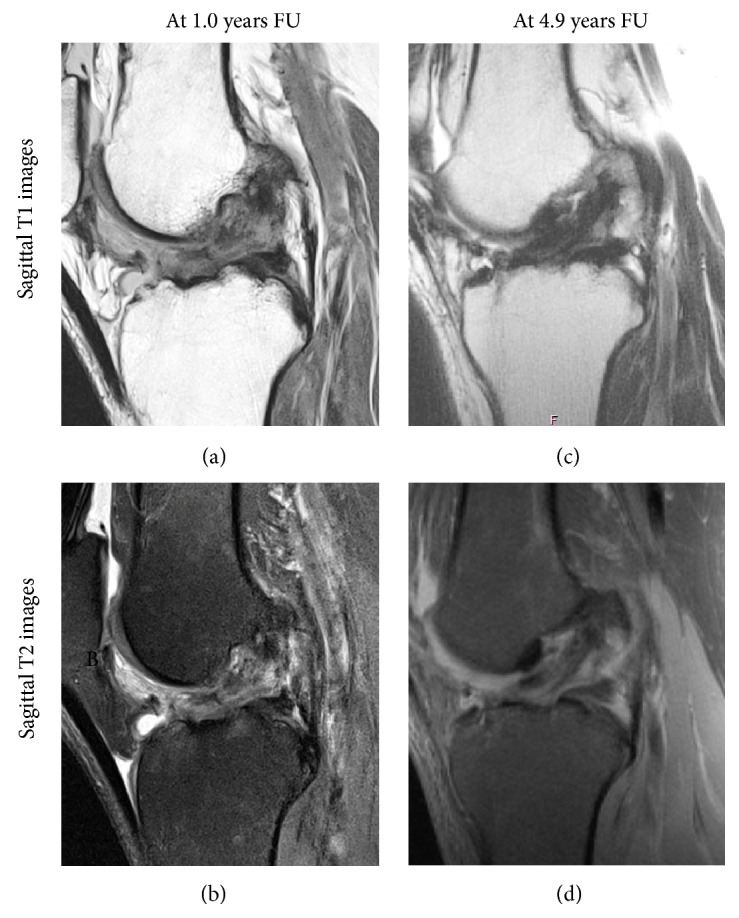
Sagittal T1 (upper row) and T2 (lower row) MR images are shown of the same patient at one and five years of follow-up. At one-year follow-up, the ligament is hyperintense and even appears to have a partial rupture of the ligament. This patient was clinically stable, and no intervention was undertaken. At five-year follow-up, the ligament on MRI was continuous and hypointense.
